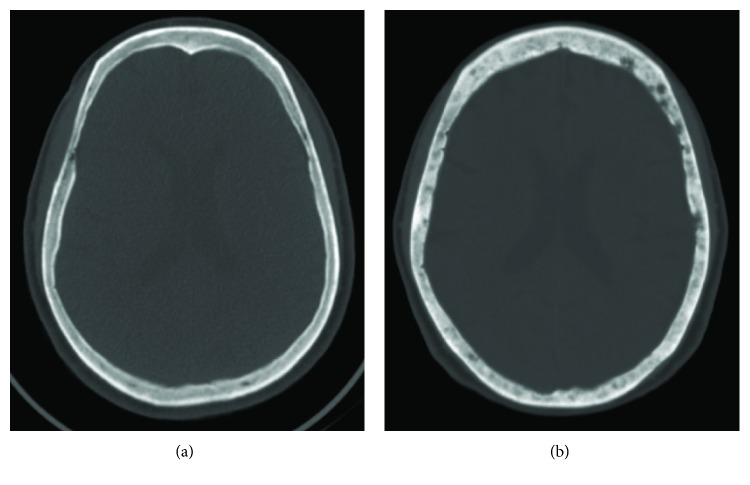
Axial head CT images on bone window. (a) The skull was intact on June 30, 2011. (b) Many osteolytic lesions were detected on September 13, 2017.
